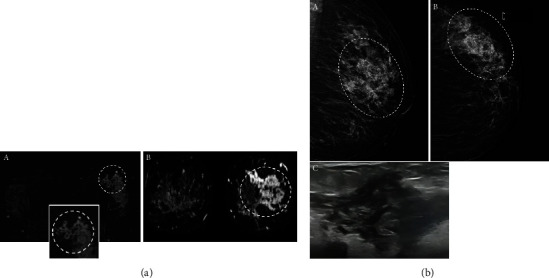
(a) Axial- and coronal-subtracted contrast-enhanced breast (A, B) MRI images in a 46-year-old female show clustered ring nonmass (dashed circle) enhancement with segmental distribution in the left breast. The cluster ring internal enhancement pattern is better visible in the inset photo. The biopsy result was invasive ductal carcinoma. (b) (A) MLO and (B) CC views of the same patient show segmental pleomorphic microcalcifications (dashed circle). (C) Targeted ultrasound demonstrates a nonmass lesion with tubular structures and architectural distortion.
